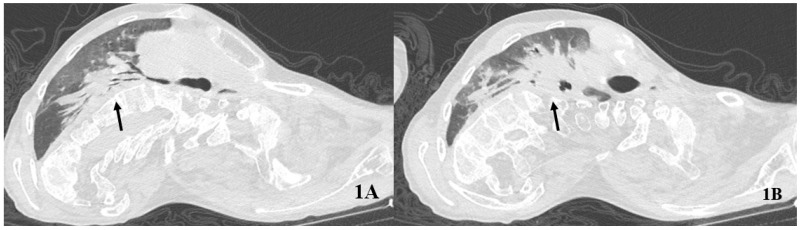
Baseline computed tomography of the chest. The arrows indicate an extensive consolidation from the right hilum (A) to the right lower lobe (B).
